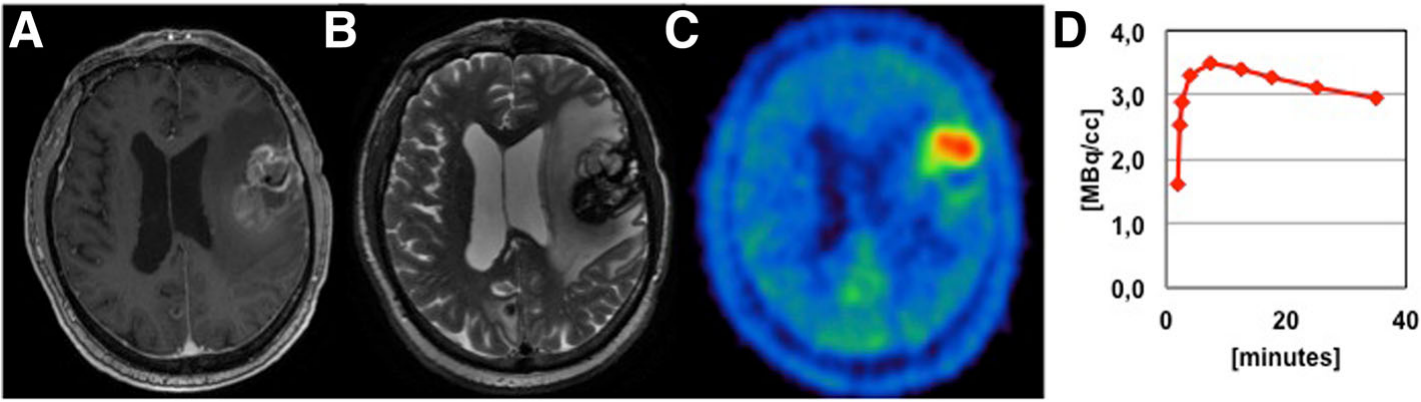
MRI and ^18^F-FET PET findings in a recurrent brain metastasis after focused high dose radiotherapy (Patient nr. 30). A ring-like contrast enhancement in the T1-weighted images (a), as well as an extensive edema in the T2-weighted images is seen (b). Both a focal ^18^F-FET uptake (TBR_max_ 3.5, TBR_mean_ 2.3) and decreasing TACs can be observed in static and dynamic PET analysis (c, d)
